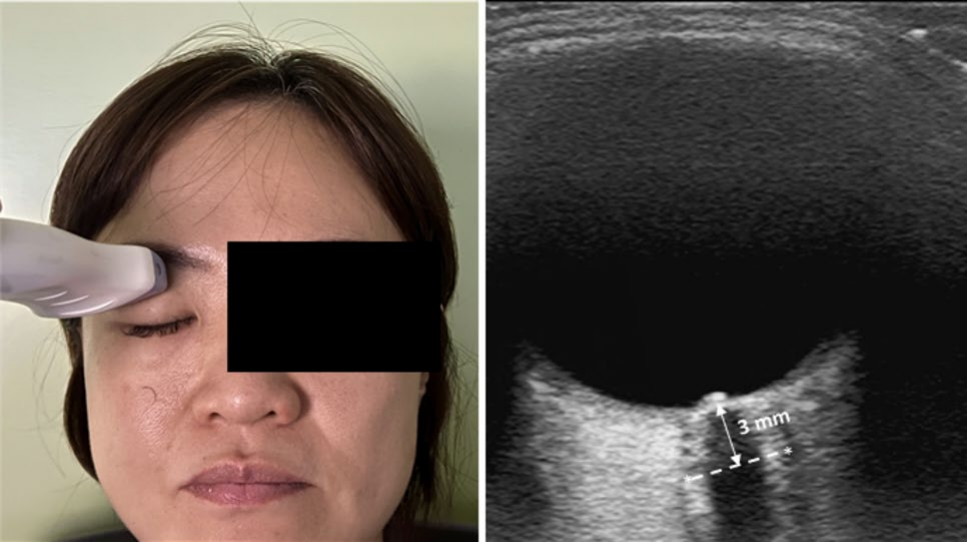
Ultrasound measurement of the optic nerve sheath diameter (ONSD). The probe was applied in the transverse plane on the eyelid, and the optic nerve was observed longitudinally behind the orbit at its widest diameter. The ONSD was assessed as the distance between the external borders of the hyperechoic area surrounding the optic nerve, 3 mm behind the retina
